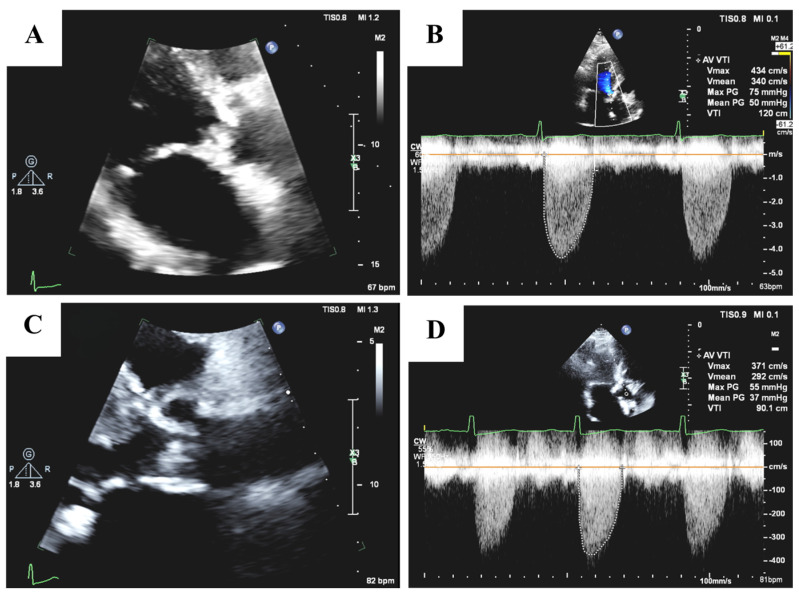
(A) Echocardiography image from a parasternal view focused on the aortic valve showing severe calcification and narrowing of the aortic valve opening. (B) Corresponding echocardiogram with key parameters, including a peak aortic jet velocity of 4.3 m/s and a mean transvalvular pressure gradient of 50 mmHg, indicating severe disease. (C) Echocardiography image from a parasternal view focused on the aortic valve showing moderate calcified aortic stenosis. (D) Corresponding echocardiogram with key parameters, including a peak aortic jet velocity of 3.7 m/s and a mean transvalvular pressure gradient of 37 mmHg, indicating moderate disease.
